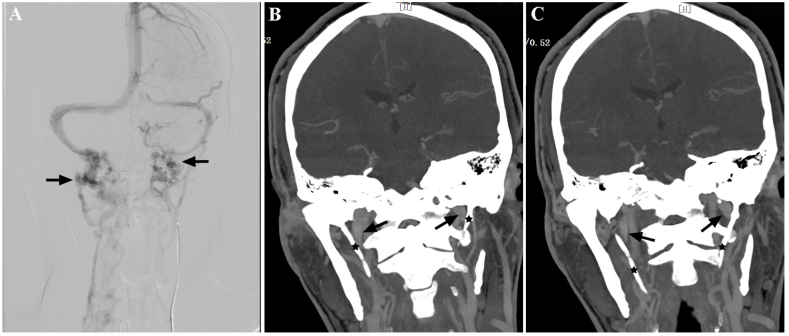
(A) Catheter angiography shows bilateral occlusion of internal jugular venous (arrows) and adjacent collaterals. (B–C) Computed tomography venography shows bilateral elongated styloid processes (asterisks) and compression of adjacent internal jugular venous (arrows).
